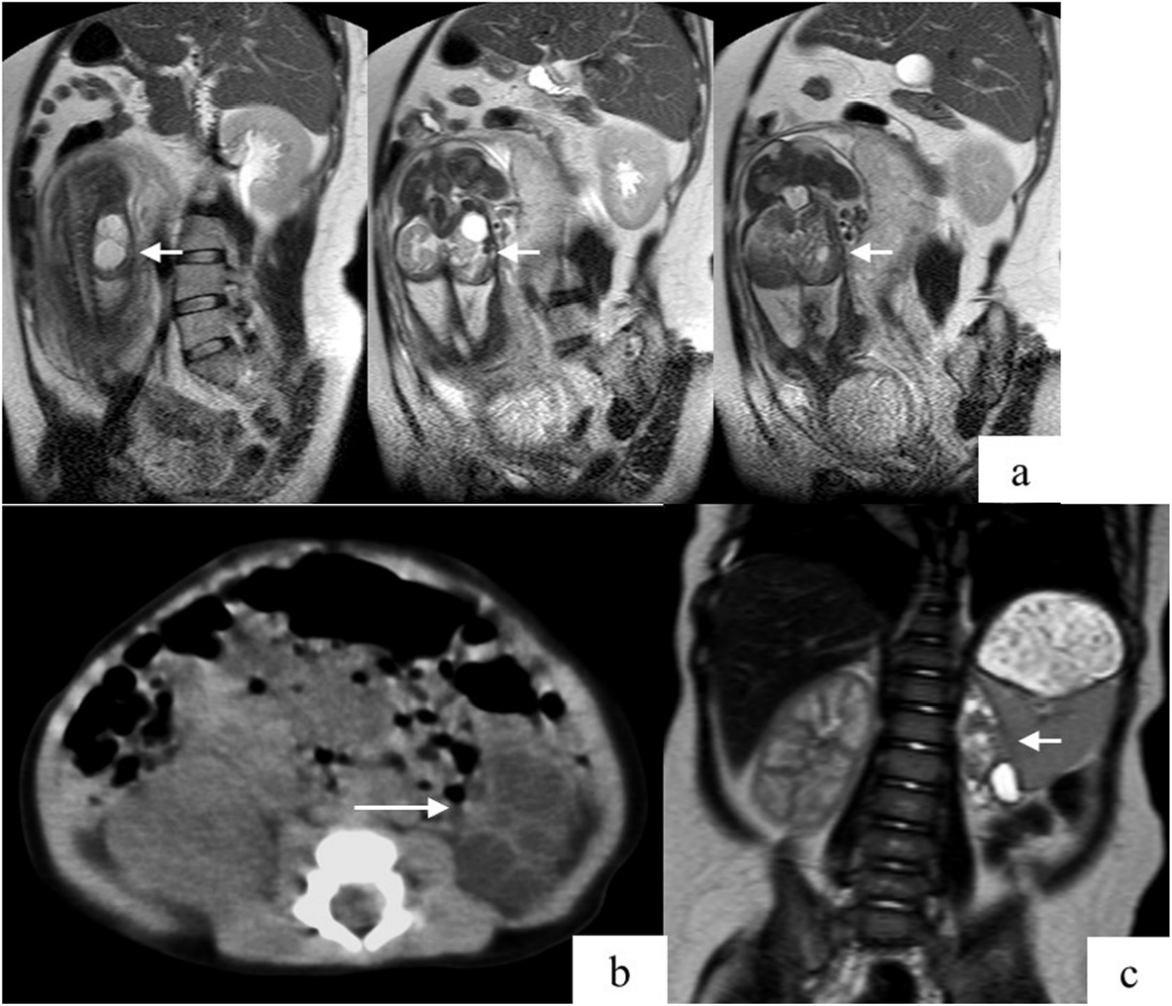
Magnetic resonance imaging. a Prenatal magnetic resonance imaging shows fetal cystic lesions of the left kidney (short arrow). b Multiple cystic lesions on the left kidney are noted on computed tomography (long arrow). c Magnetic resonance imaging shows atrophic left kidney with multiple cysts (short arrow)
